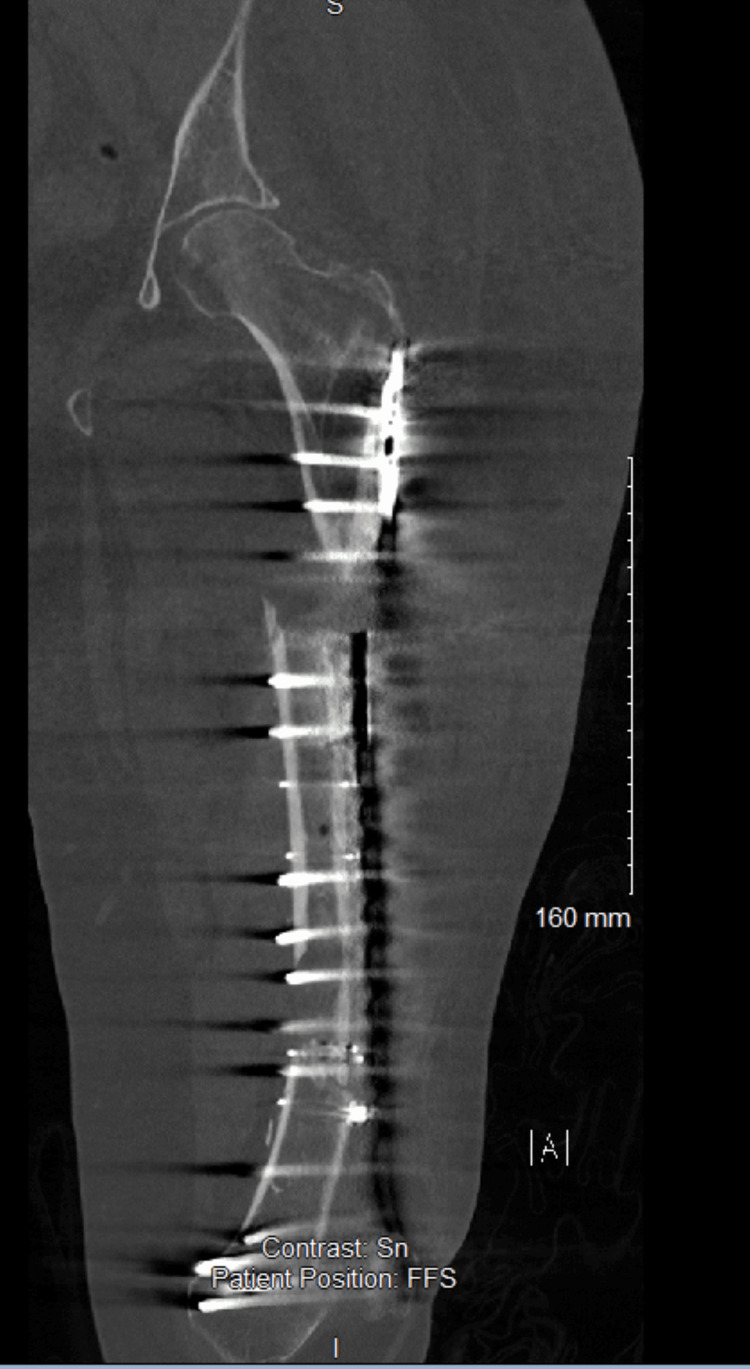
Coronal view of the computed tomography scan of the left femur on arrival at our hospital
